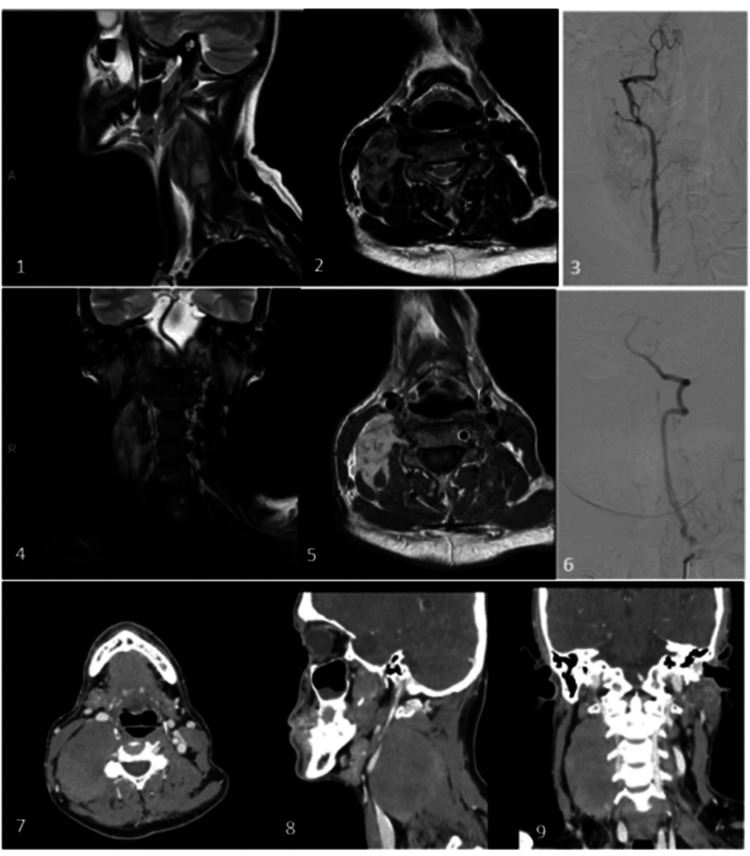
Patient's radiologic images Magnetic resonance imaging (MRI) shows a tumor next to the right levator scapulae, scalenus medius, and paravertebral muscles at levels C2 to C6 on the T2 sequence (1 and 2). It is closely related to the patient’s right vertebral artery (4) and presents an infiltrative pattern in the C3-4 and C4-5 foramina and adjacent muscles on the T1-weighted sequence (5). Vertebral artery angiography found segmental hypoplasia of the right vertebral artery after the posterior inferior cerebellar artery (PICA) branching (3) and significant atheromatous stenosis of the left vertebral artery at its origin (6), resulting in improbable compensation from the left side (negative right occlusion test). A CT scan revealed a tumor in the right paravertebral region (7) in contact with the internal jugular vein and no signs of infiltration (8); a cleavage space is maintained between the right sternocleidomastoid (SCM) muscle and the tumor (9).
